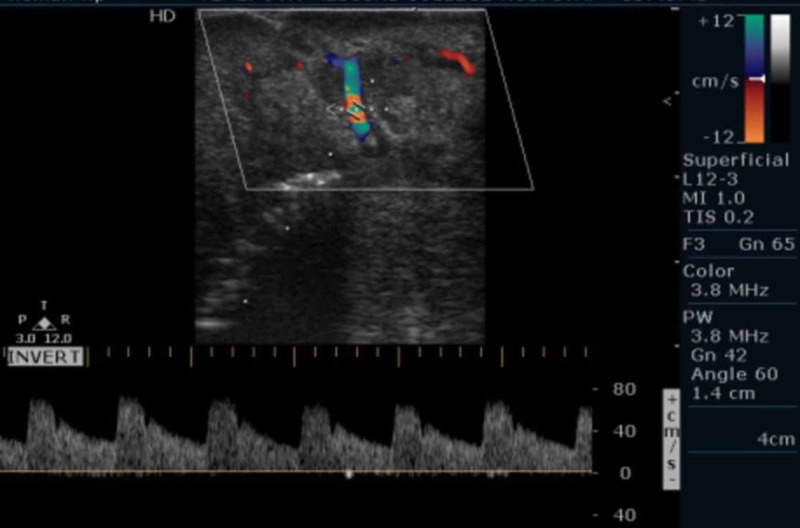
Color doppler application on USG showing turbulence of blood flow signals on the bottom suggesting AVM.‎ USG, ultrasonography; AVM, arterio‎venous malformation
